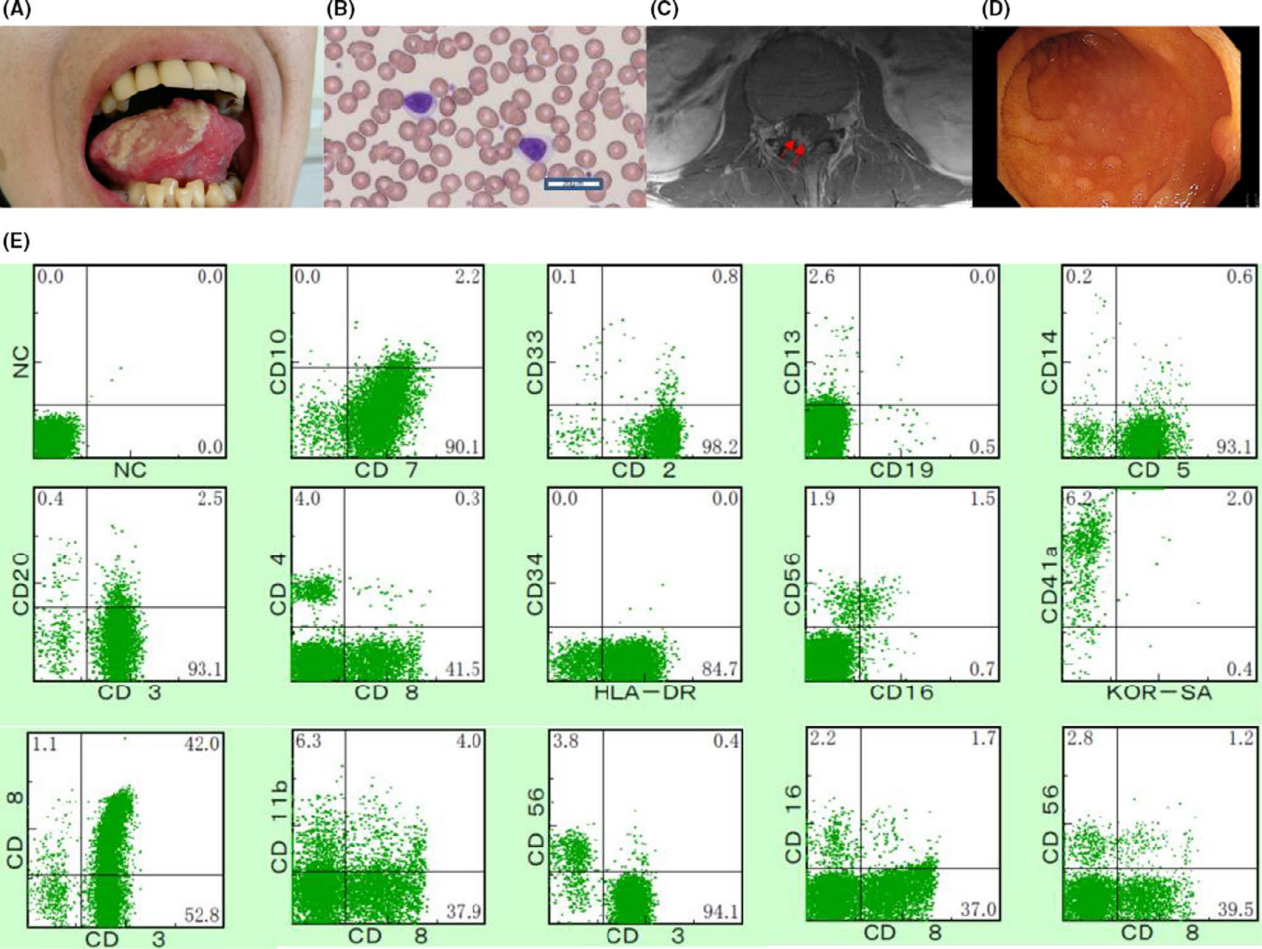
A, Refractory oral ulcers. B, Peripheral blood smear of leukemic cells. C, Flow cytometric analysis of the bone marrow. D, MRI with contrast of the lumbar. (arrow: enhanced lesion in the L4‐S1 nerve root). E, Colon ulcers
